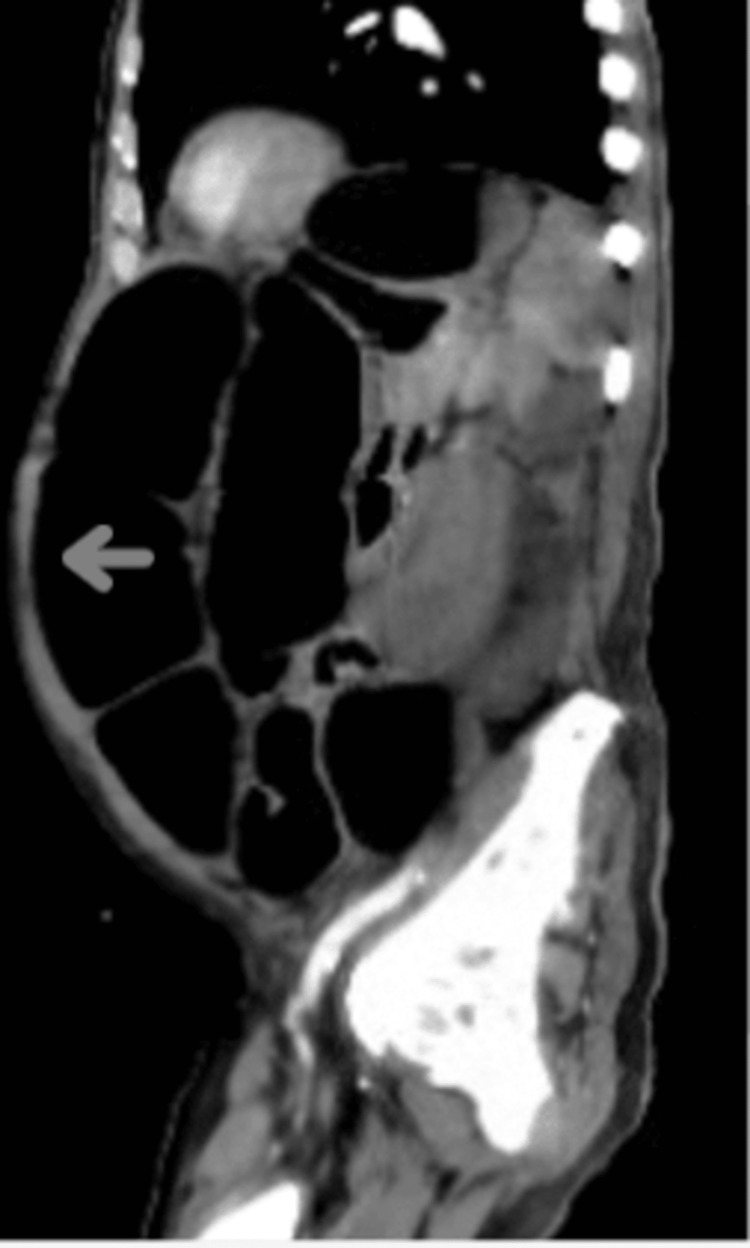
CT scan sagittal view showing marked gaseous distention of the colon representing megacolon. Marked gaseous distention of the colon, which may represent a megacolon. No obvious obstructing lesion is identified.
